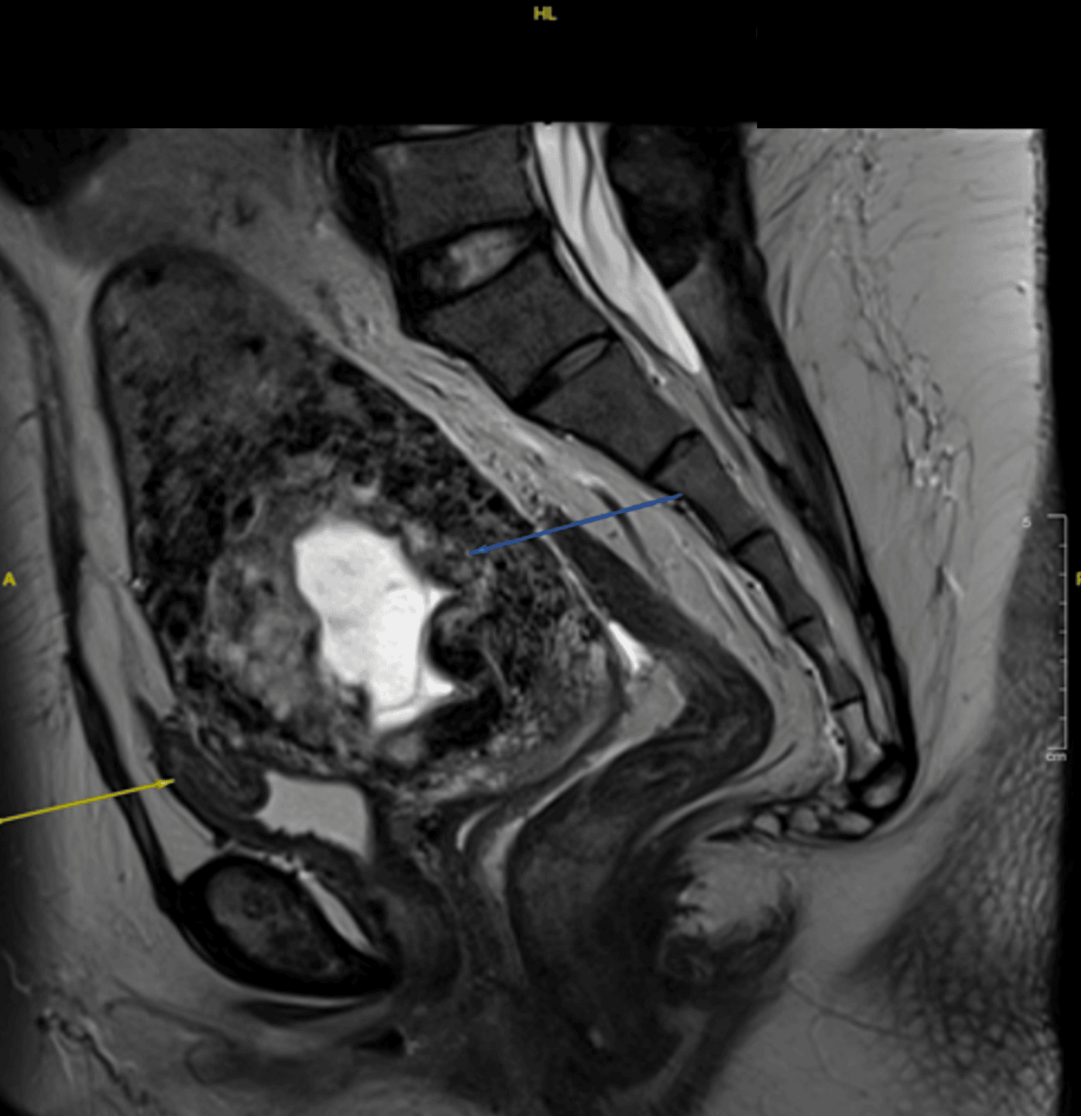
MRI upon completion of chemotherapy showed a stable partially invasive molar pregnancy breaching through the cesarean scar (blue arrow) with urinary bladder invasion (yellow arrow)
